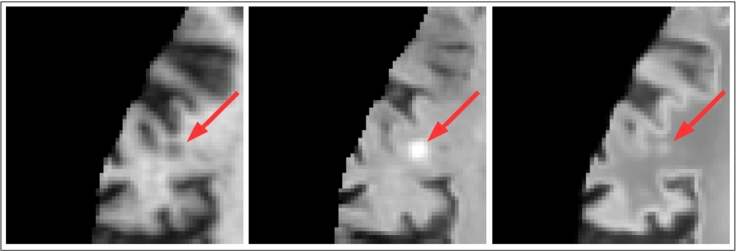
A case where a lesion close to the cortex is mistakenly synthesised as hyper-intense. Left: *T*_1_-w image. Middle: FLAIR image. Right: Corresponding synthetic FLAIR image.
